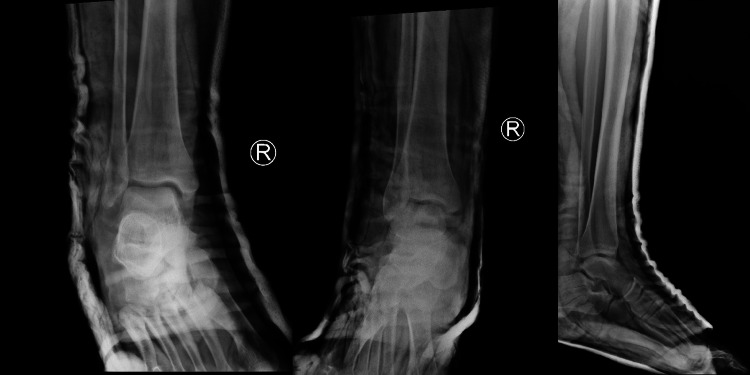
Post reduction radiographs of ankle.
